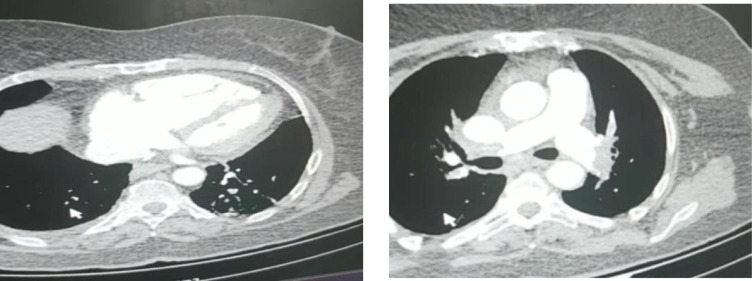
Pulmonary computed tomography angiography of case 1
